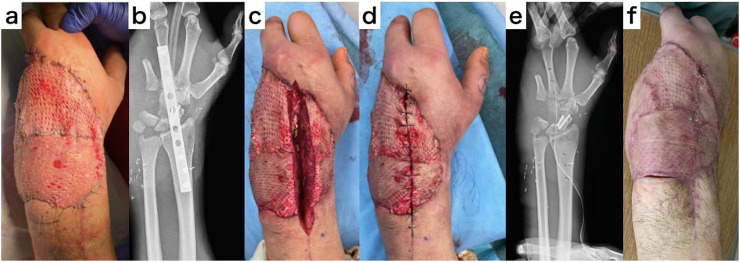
Treatment course of case 1. a. Appearance before secondary surgery. b. X-ray before secondary surgery. c. Appearance after provisional incision. d. Appearance after rough suture. e. X-ray after secondary surgery. f. Appearance after wound healing.
